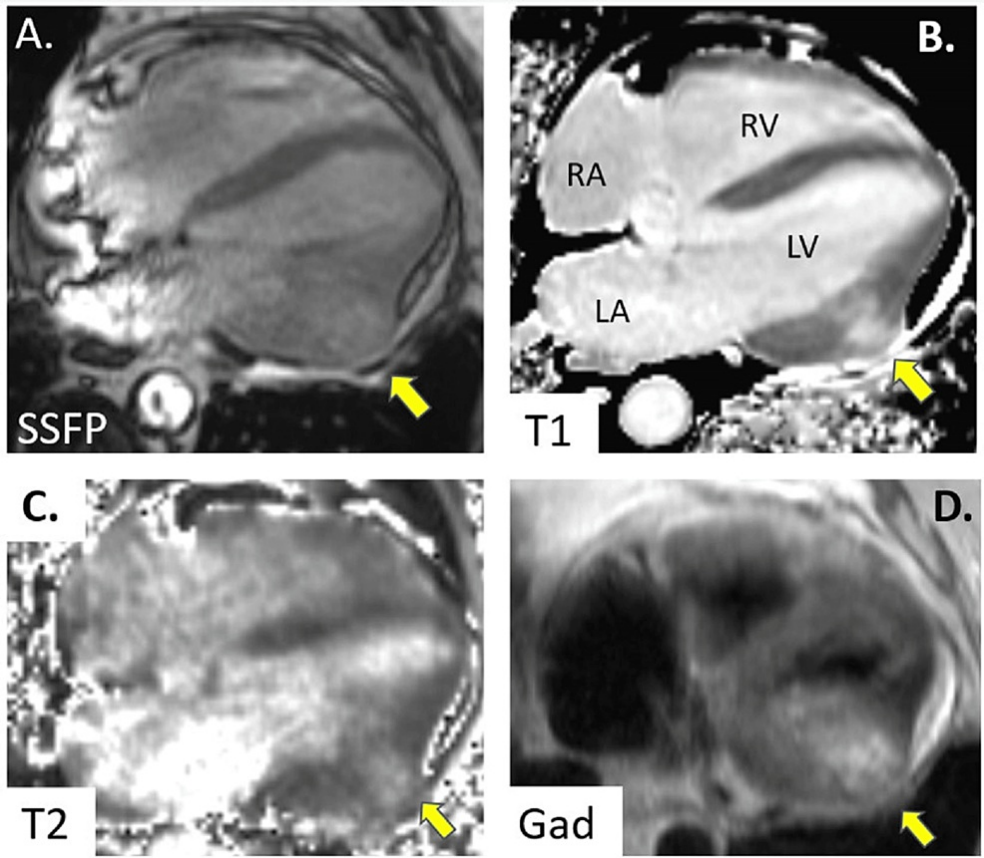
Cardiac magnetic resonance imaging Panel A: Steady-state free precession (SSFP) gradient echo image shows a bulging mass in the mid-lateral wall of the left ventricle. Panels B-D: T1 and T2 MyoMaps show increased native T1 and T2 relaxation times with late gadolinium (Gad) enhancement, suggesting increased extracellular space and edema in the mass. LV - left ventricle, RV - right ventricle, LA - left atrium, RA - right atrium
